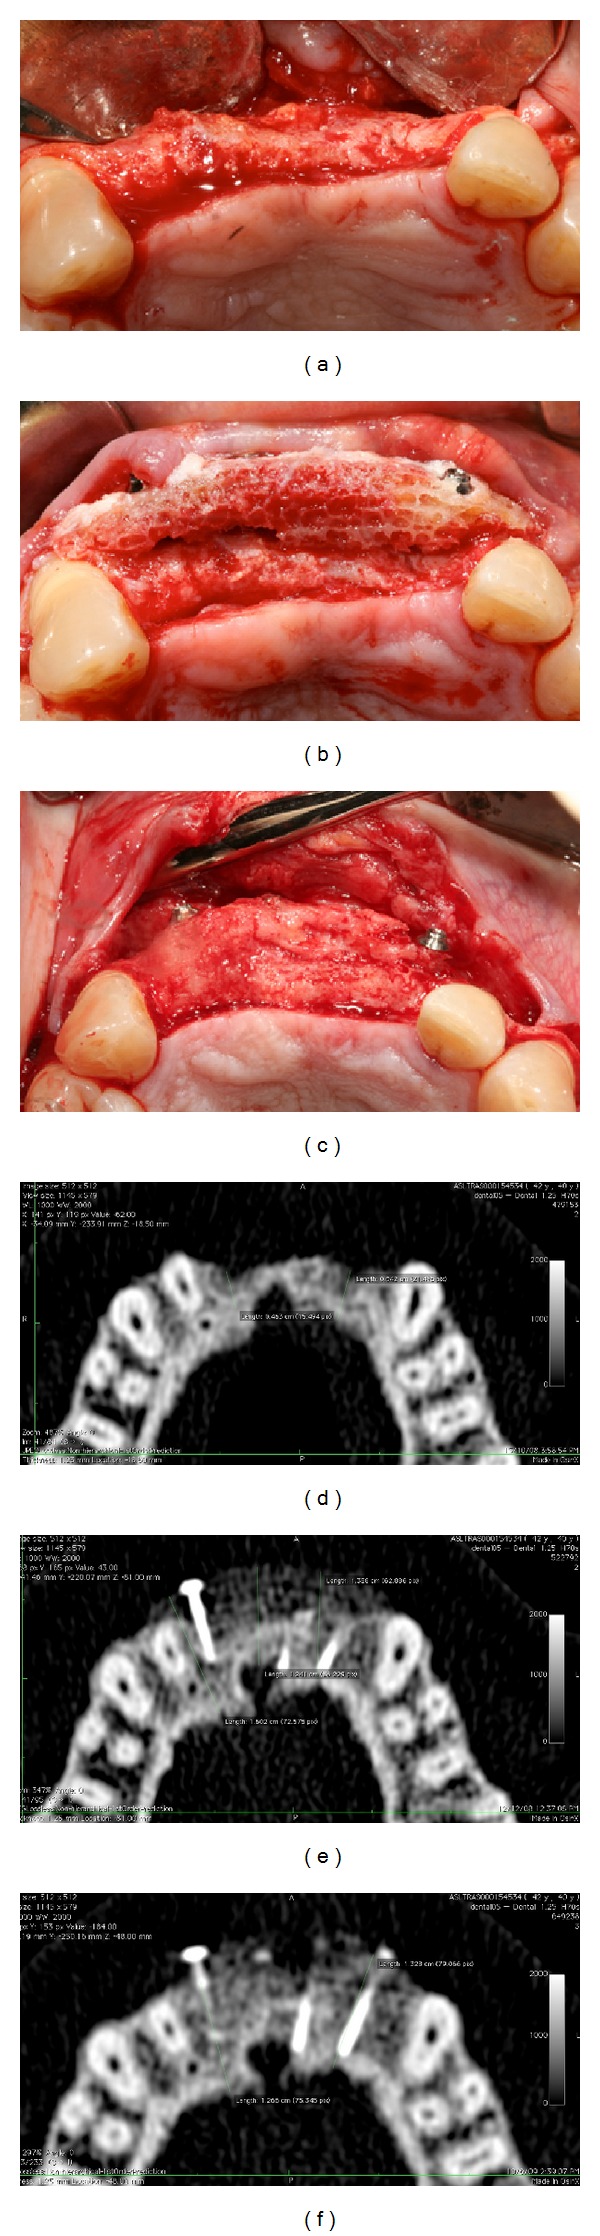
(a) Atrophic ridge before grafting; (b) homologous bone graft in place and (c) after 6 months of healing during surgery for implant placement. CT scans were taken before the intervention (d), 1 week after surgery (e), and after six months of healing (f).
